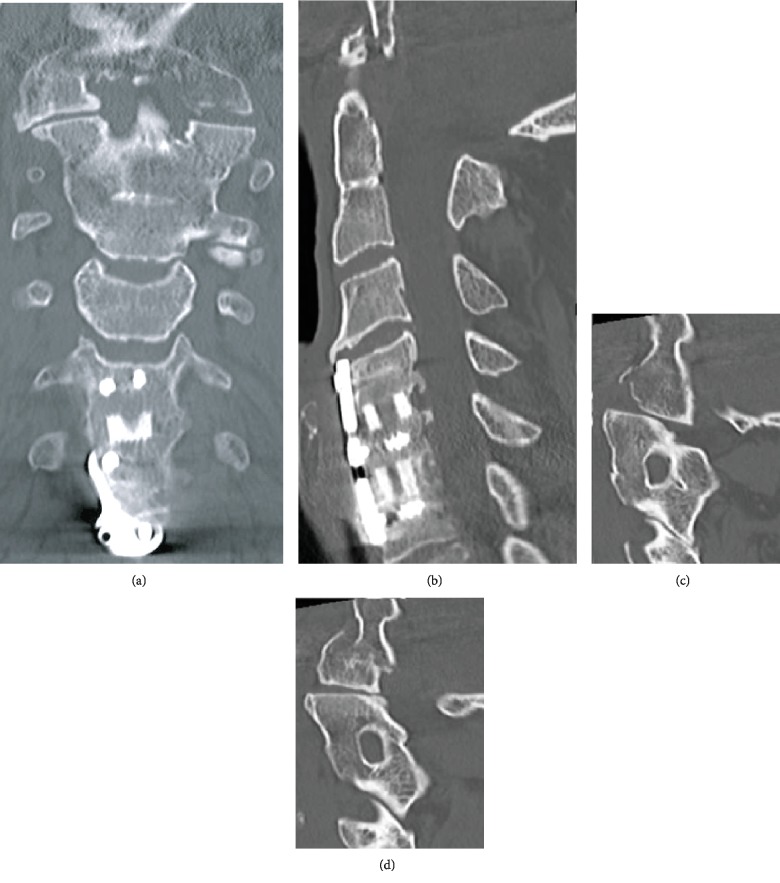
Case 1: cervical CT. (a) Complete C1 assimilation, C2-C3 congenital fusion block. (b) Previous C5-C7 ACDF. The C1 anterior ring is fracture displaced and positioned anterior to the clivus. The C1 posterior arch is fused to the occiput. (c) Left atlantoaxial subluxation. (d) Right condyle C1 lateral mass fracture dislocation.
